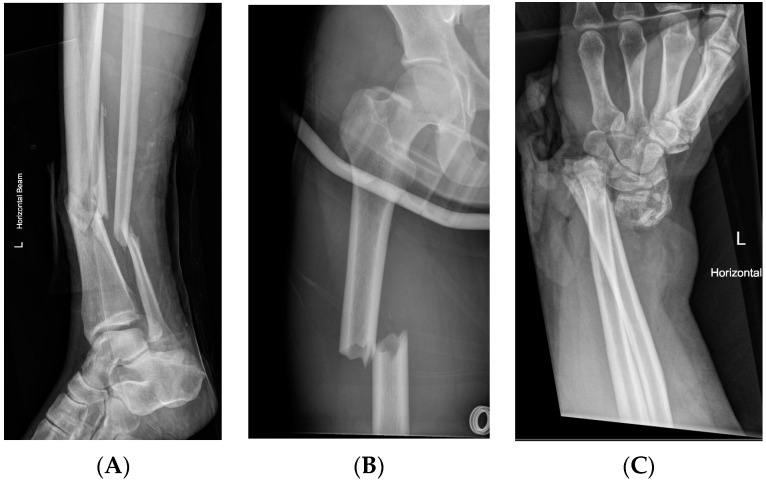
Plain films demonstrating high-energy nature of e-scooter-related orthopaedic injuries. (A) Open tibial and fibula diaphyseal fractures; (B) Midshaft femoral fracture; and (C) Open distal radius fracture.
